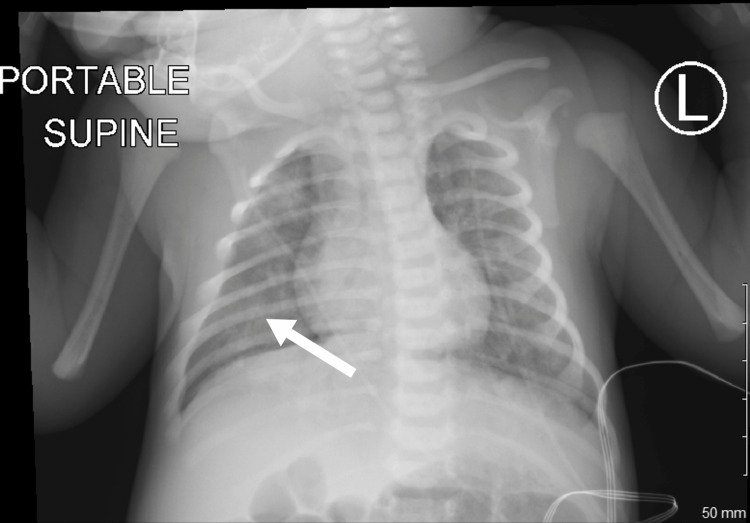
Chest X-ray on day of life 2, significant for bilateral infiltrates, right (white arrow) greater than left
